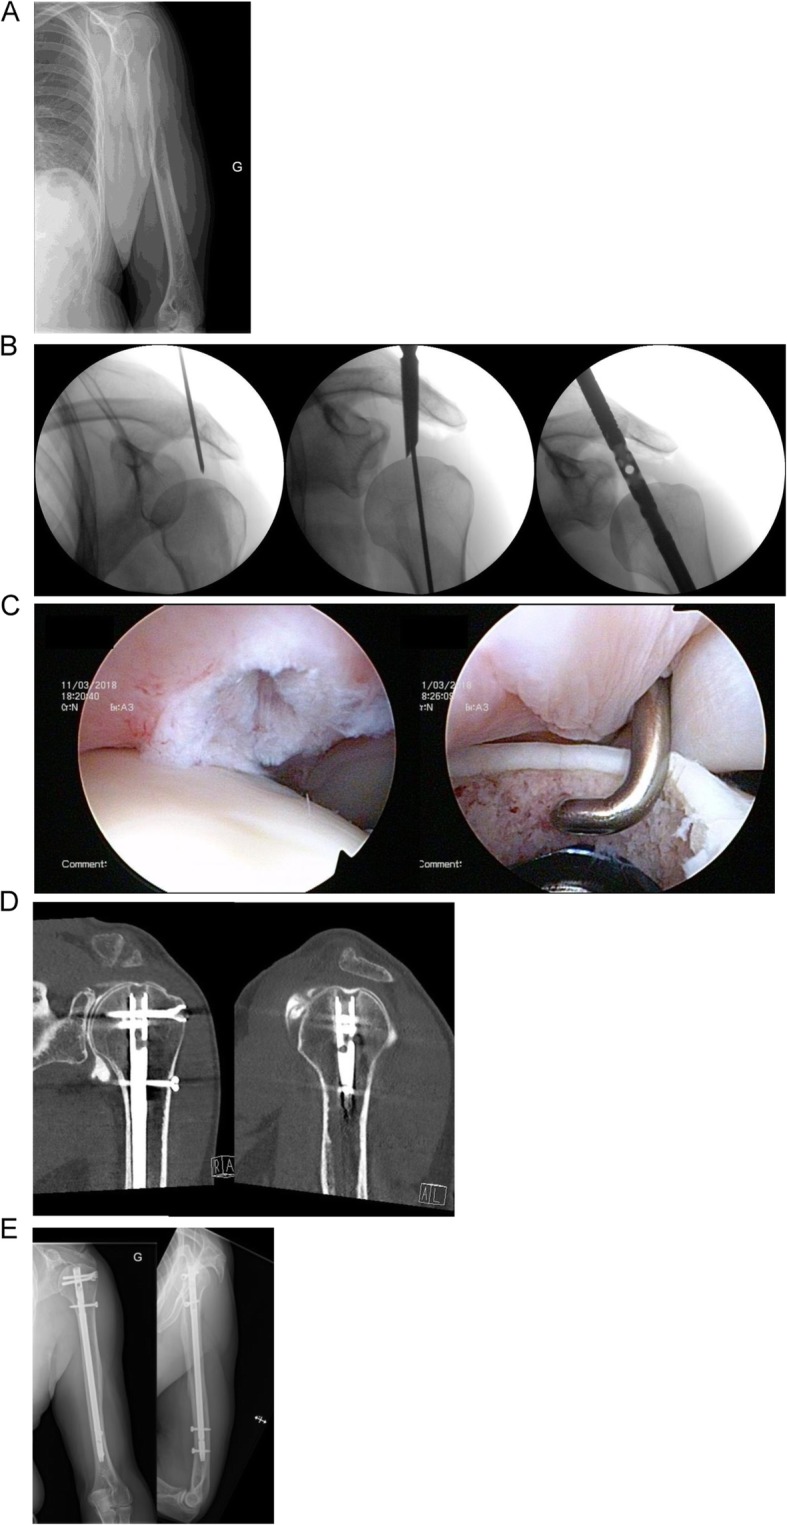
Male patient, 57 years, sustained a diaphyseal spiral fracture after a fall from stairs (1a). Three intraoperative views with the image intensifier: insertion point and direction was determined using a 2.0 mm Kirschner wire. After the feasibility has been verified a cannulated awl is used to perforate the supraspinatus tendon and the articular surface. With the awl in situ the Kirschner is removed and replaced by a long guide wire which then directs the nail (1b). After stabilisation a diagnostic arthroscopy is performed to document the perforation of the rotator cuff, the appropriate depth of the nail and to exclude concomitant intraarticular lesions (1c). Five months after surgery a CT with intraarticular application of contrast medium was performed; a leakage or lesion to the rotator cuff was excluded (1d). Bony consolidation at 6 months after surgery (1e)
